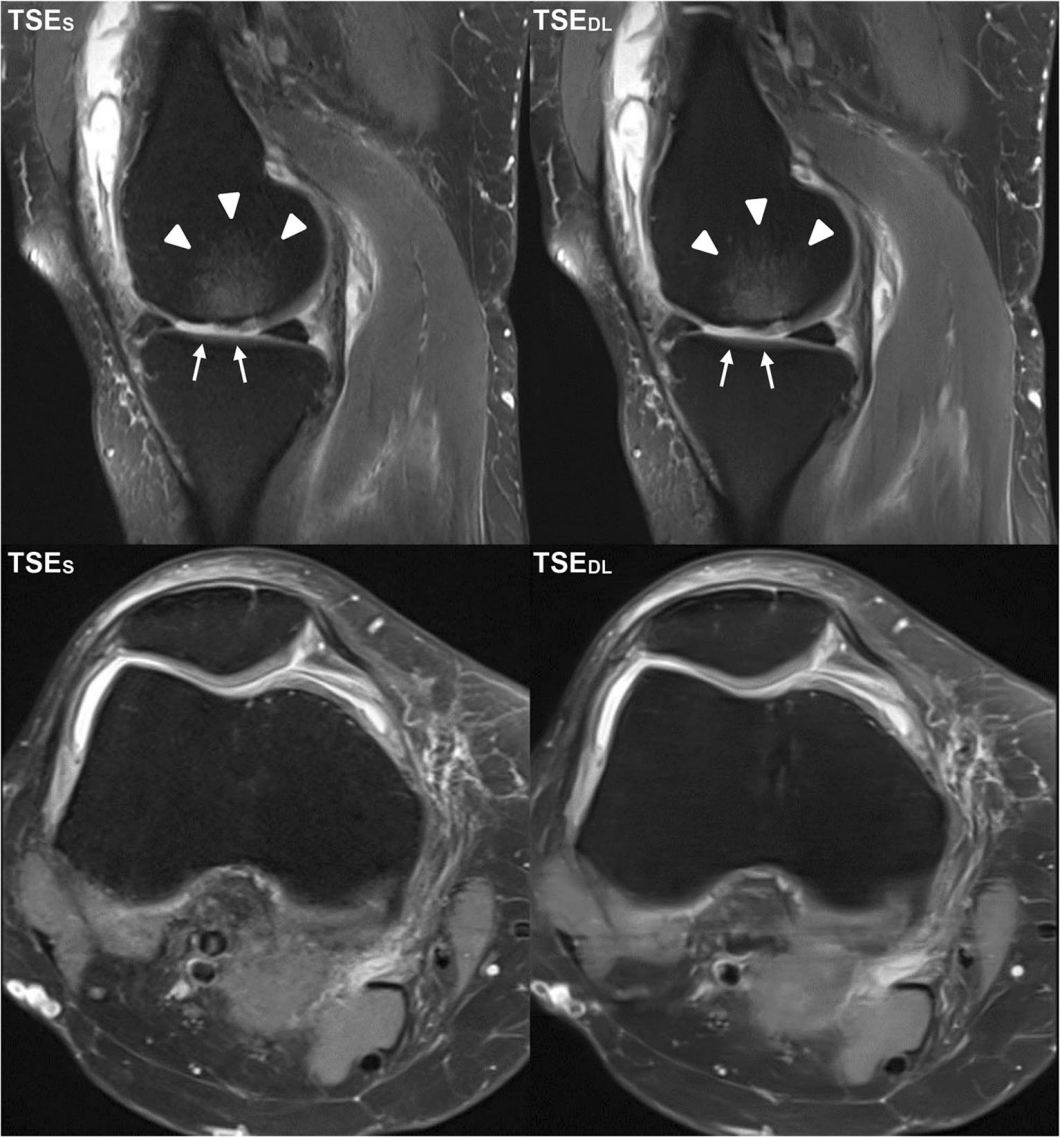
Image example of a standard and deep-learning-reconstructed TSE imaging of the knee at 1.5 T. This is an example of a knee MRI at 1.5 T in sagittal and axial orientation of a 52-year-old patient with pain in the medial side of the right knee. In the sagittal images, the cartilage defect (ICRS grade 4; white arrows) of the medial femoral condyle with adjacent bone marrow edema (white arrowheads) is visible in both TSE_S_ (left) and TSE_DL_ (right). Comparing TSE_S_ (left) and TSE_DL_ (right) especially in the axial orientation, TSE_DL_ shows characteristic banding artifacts of deep-learning-accelerated images when acquired at 1.5 T
